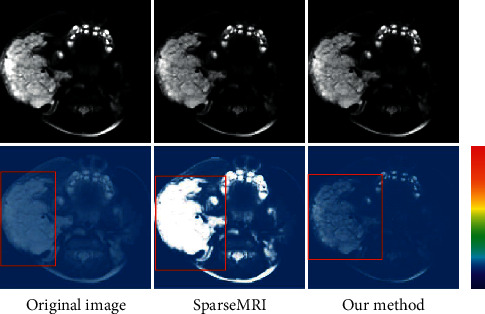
MRI image and residual image after reconstruction with different algorithms.
